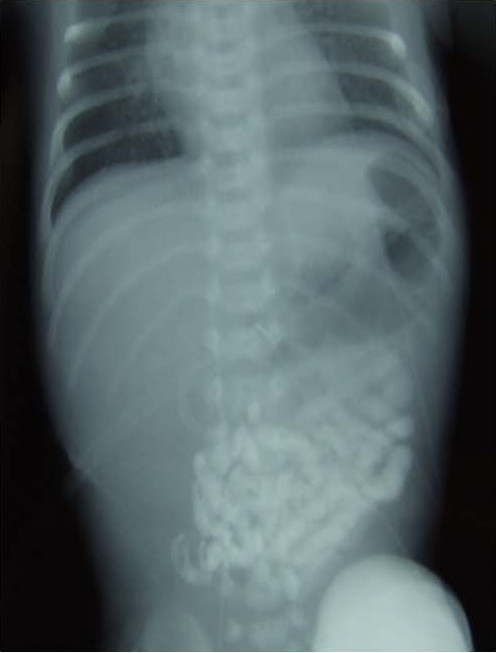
Postoperative abdominal X-Ray with barium meal.
